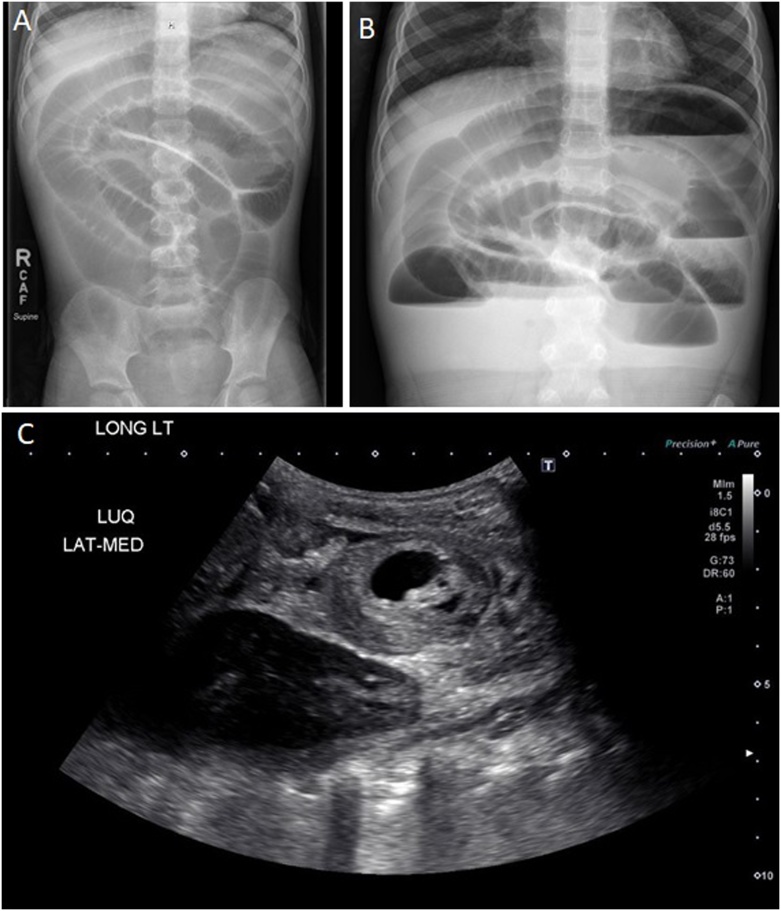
Preoperative imaging. Panel A: antero-posterior abdominal X-ray in decubitus position Panel B: antero-posterior abdominal X-ray in standing position; Panel C: abdominal ultrasound with the arrow pointing at the intussusception.
